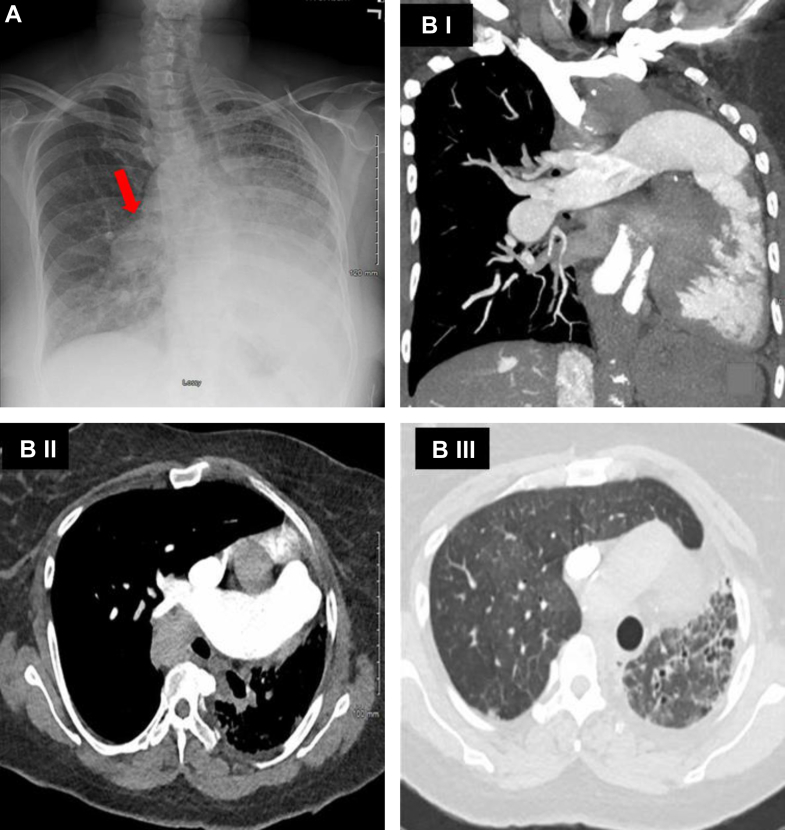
Chest X-Ray and CT Chest With Contrast Chest x-ray (A). The red arrow highlights a right descending pulmonary artery. A finding associated with pulmonary hypertension. Computed tomography chest with contrast (B). Coronal view demonstrating the absence of the left pulmonary artery, enlargement of the main and right pulmonary arteries without pulmonary embolism, hyperinflation of the right lung, and hypoplasia of the left lung (B.I). Transverse view of the chest (B.II). Another transverse view demonstrating hyperinflation of the right lung and hypoplasia of the left lung (B.III).
